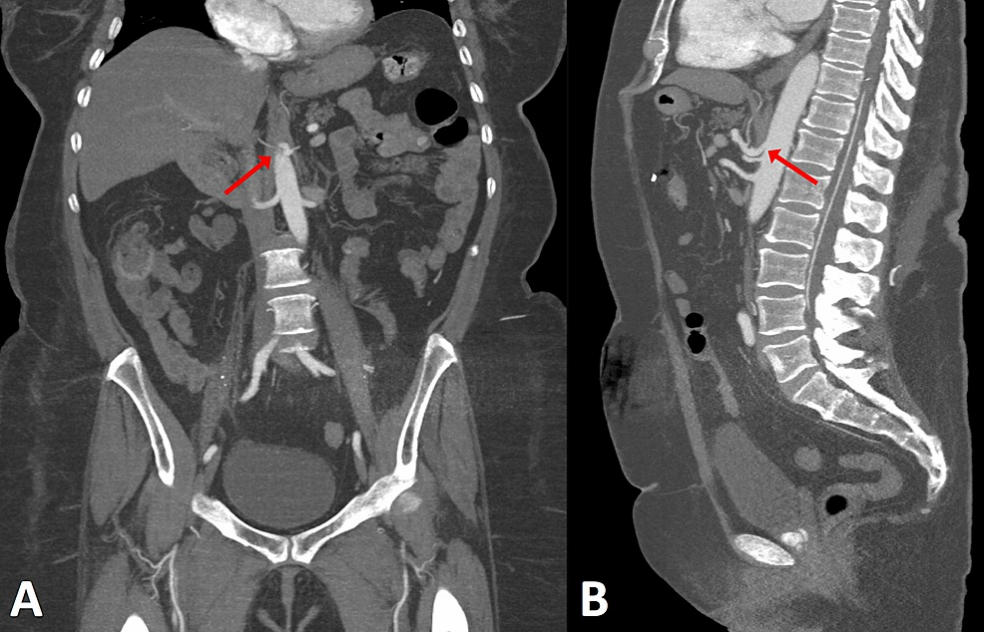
CT imaging of celiac artery stenosis (arrows) in the coronal (A) and sagittal (B) planes. CT, computed tomography
